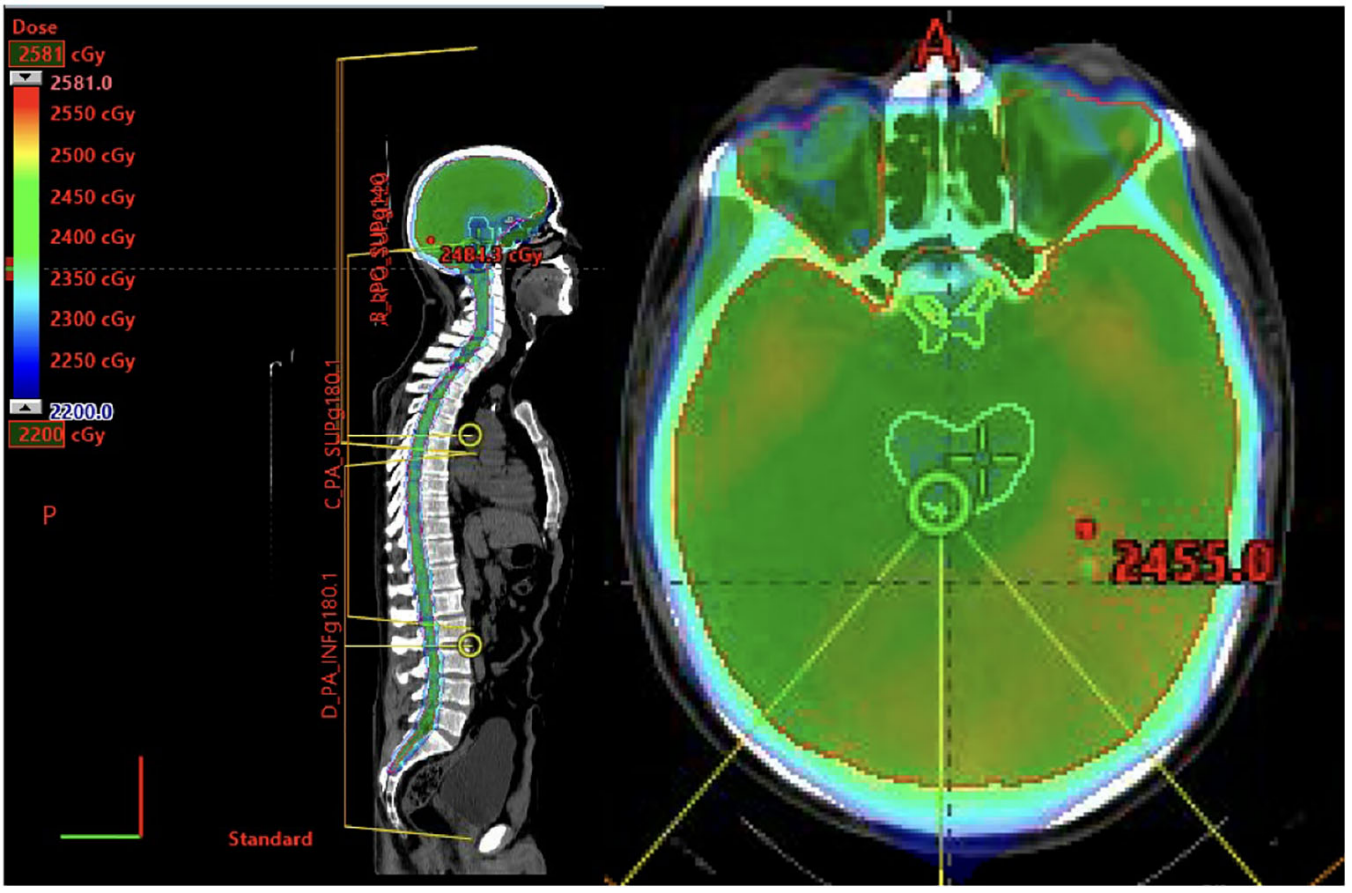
42-year-old Male with Philadelphia chromosome positive B-cell ALL s/p hyper CVAD, TKI, and full-dose TBI followed by stem cell transplant. One year later he experienced an isolated CNS relapsed diagnosed on lumbar puncture and MRI showing radiographic optic nerve involvement. He cleared his CSF s/p hyper-CVAD and IT cytarabine. He received consolidative RT with proton craniospinal irradiation to 23.4 Gy in 13 fractions.
